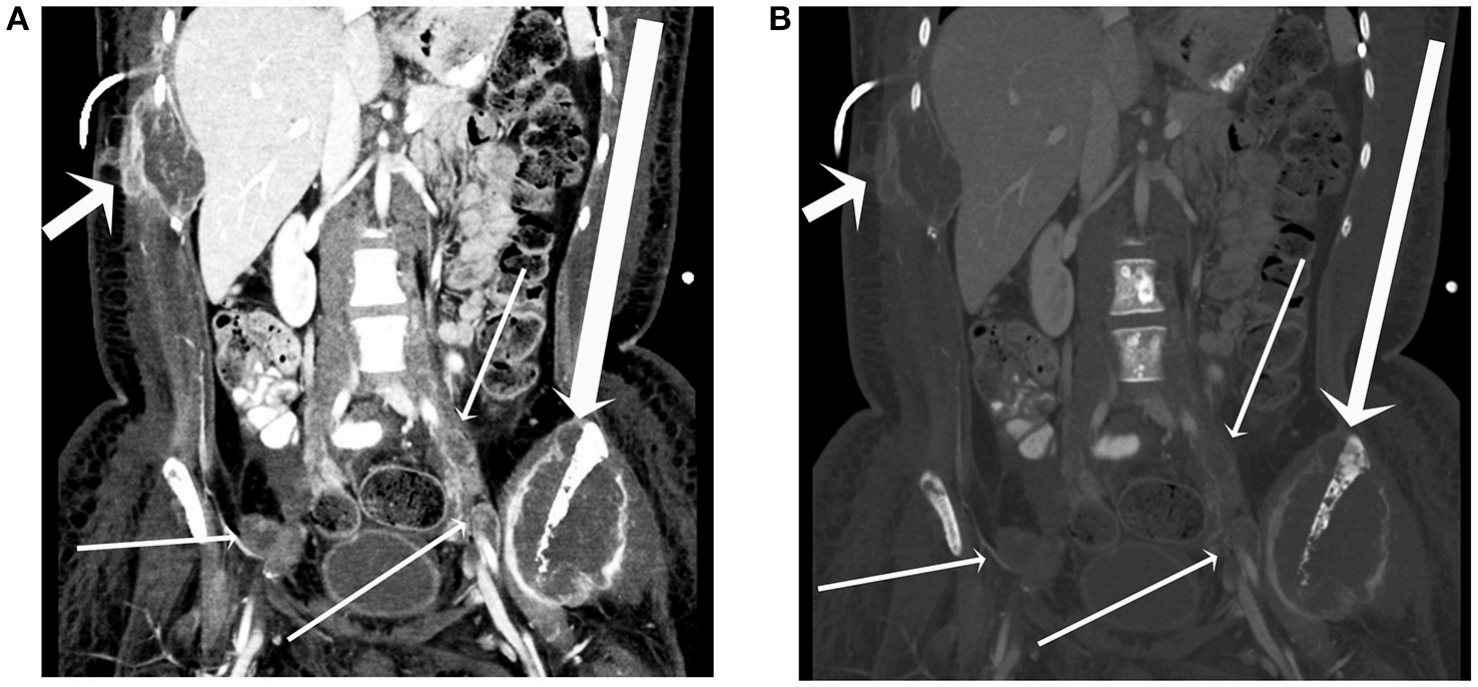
A 17-year-old female with H3F3A G34R mutated infiltrative glioma. A coronal image from a CT of the abdomen and pelvis with contrast in soft tissue window (A) and bone window (B) reveals a destructive lesion of the left iliac bone with large soft tissue component (long arrow), a destructive lesion centered around a right lower rib adjacent to a plural drainage catheter (short arrow), and iliac lymph node metastases (thin arrows).
